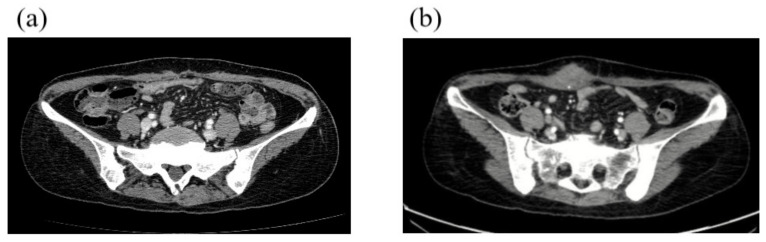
Imaging of patient No 1. in 2019. (a) Computed tomography (CT) scan of the pelvis showed a metastatic lesion in the rectus muscle in January 2019. (b) The metastatic lesion in the rectus muscle had increased in size by March 2019.
